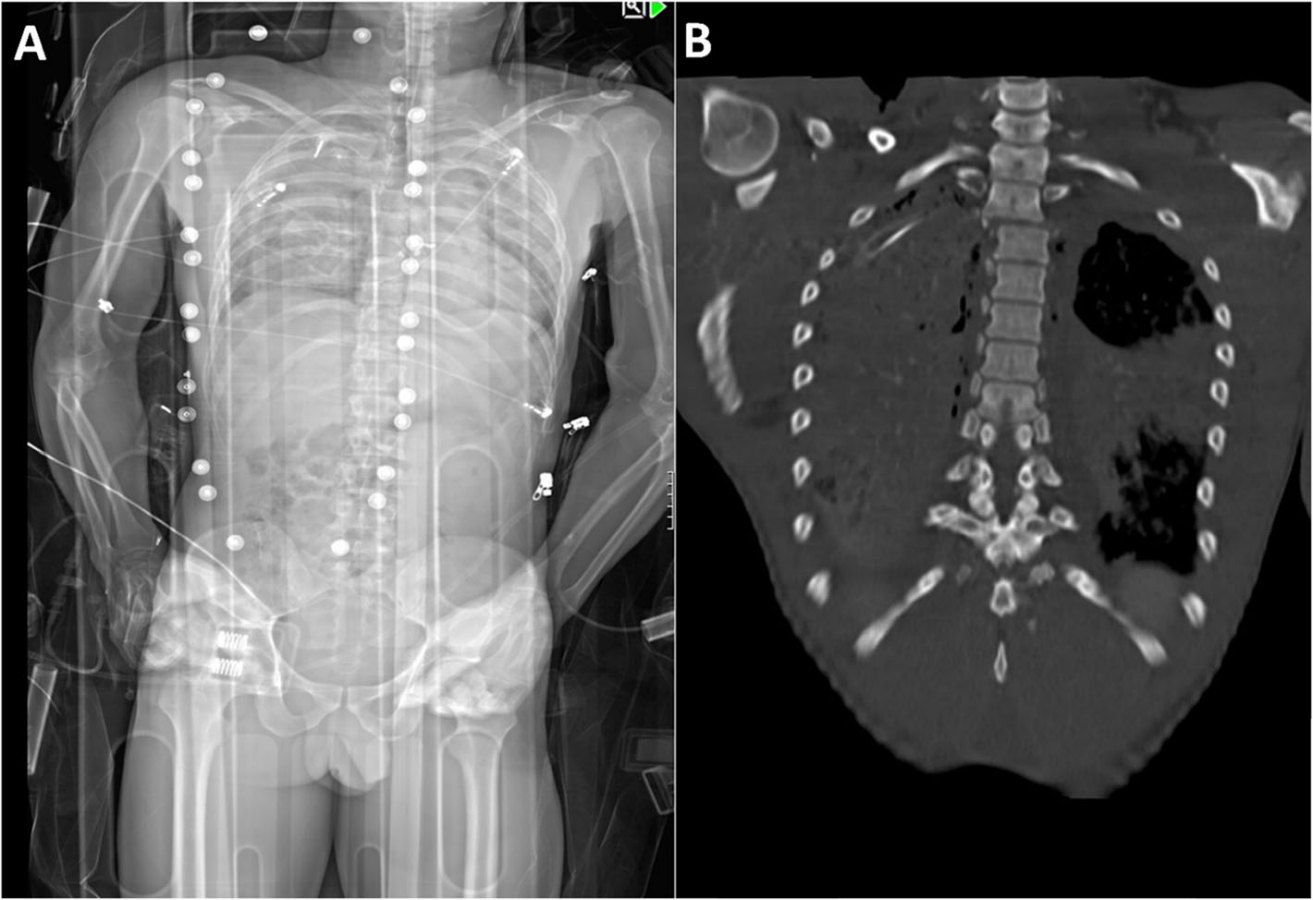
Example of a missed unstable injury using anterior-posterior Lodox Statscan (AP-LS). An unstable spinal injury fo the second and third thoracic vertebra shown in AP-LS (a), and the full-body CT scan (b) of 46-year-old men after falling from a height. None of the three observers identified the injury in AP-LS
